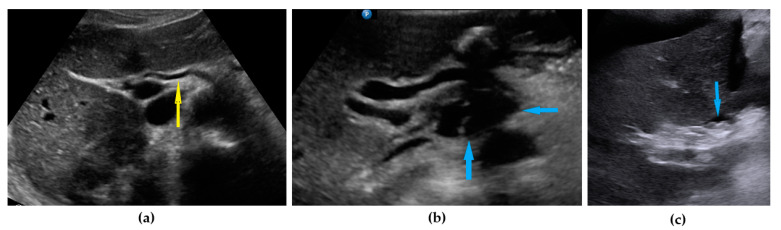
Ultrasound images of hepatic hilum: (a) normal hilum (yellow arrow identify hepatic artery); (b) tumor under hepatic artery; (c) carcinomatosis on the peritoneum around hepatic hilum. Blue arrows identify tumors.
